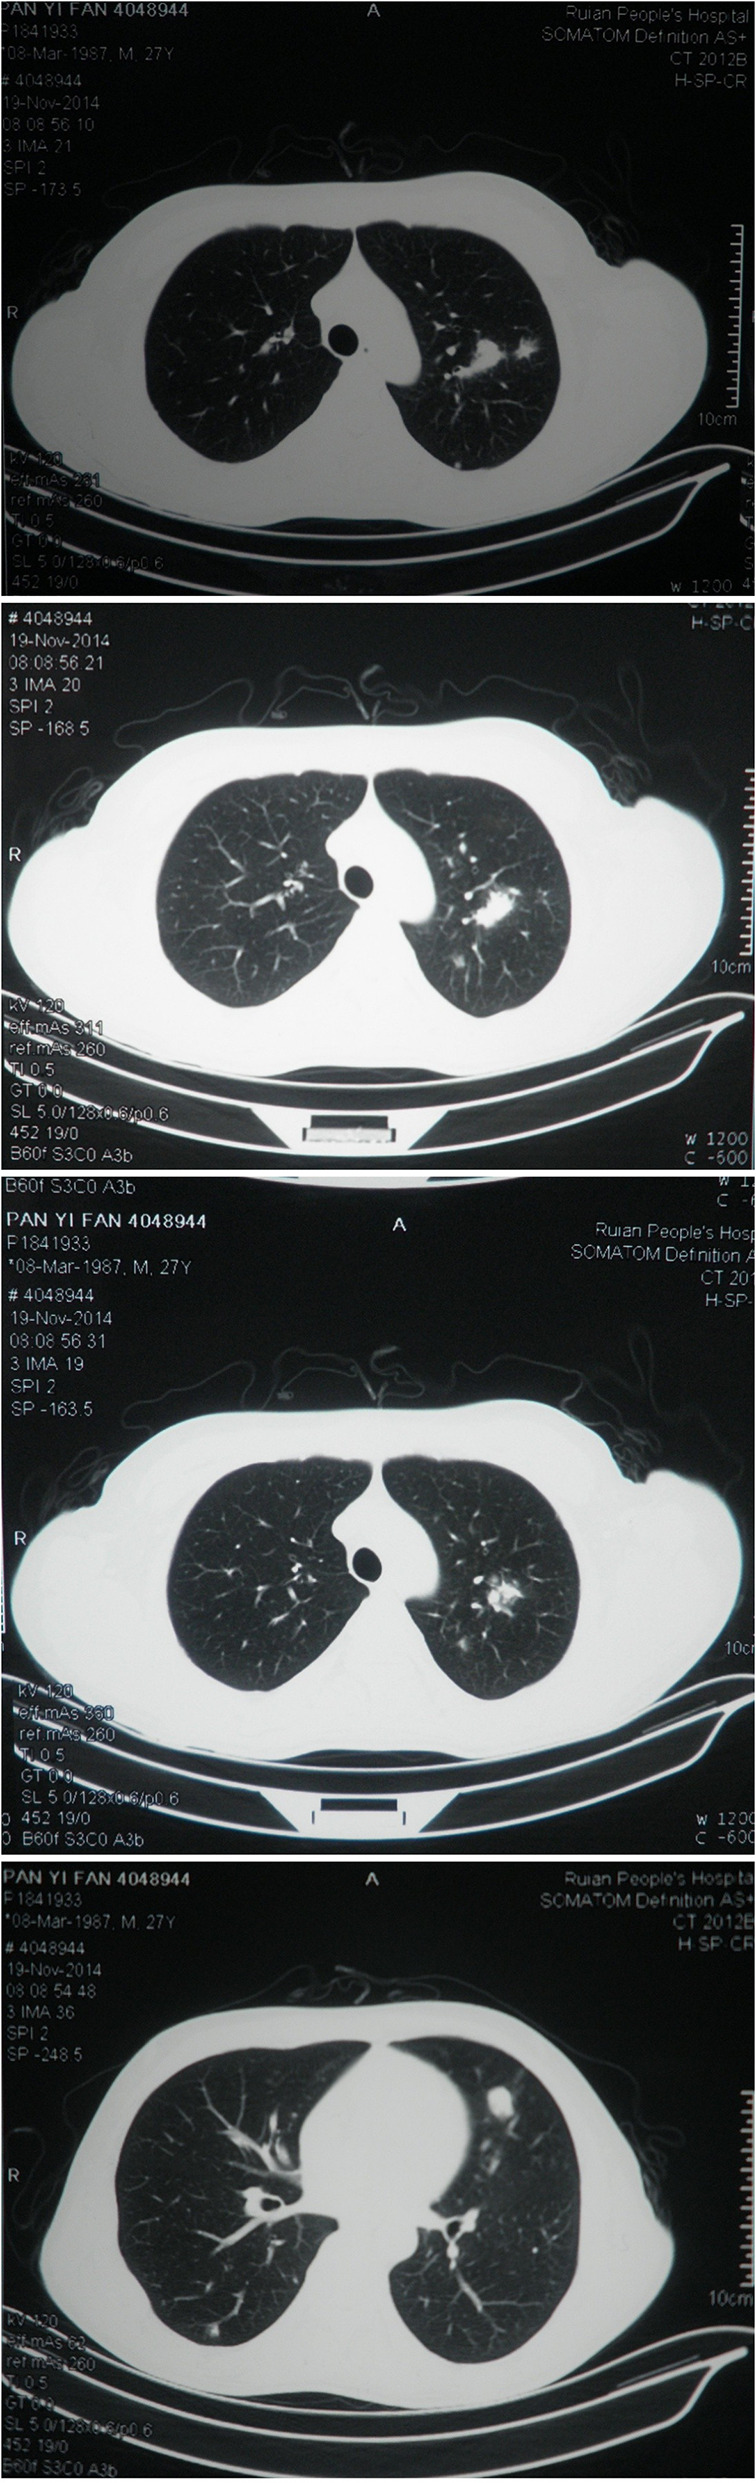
Pulmonary CT scan showed the multi-transfer lesions of lung at the 11th month of onset.
